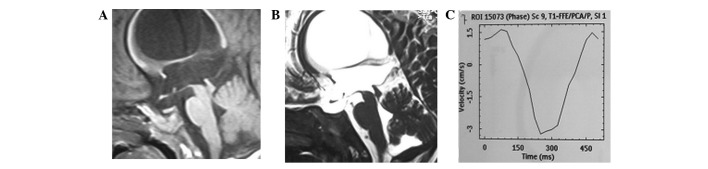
Magnetic resonance imaging (MRI) of a patient one year after surgery shown in: (A) sagittal T1-weighted MR image; the cerebrospinal fluid (CSF) flow-void sign within the aqueduct and fourth ventricle were not observed; (B) sagittal T2-weighted MR image revealed re-occlusion of the aqueduct. (C) CSF flow waveform showing a flow pattern revealed that the aqueduct was open.
